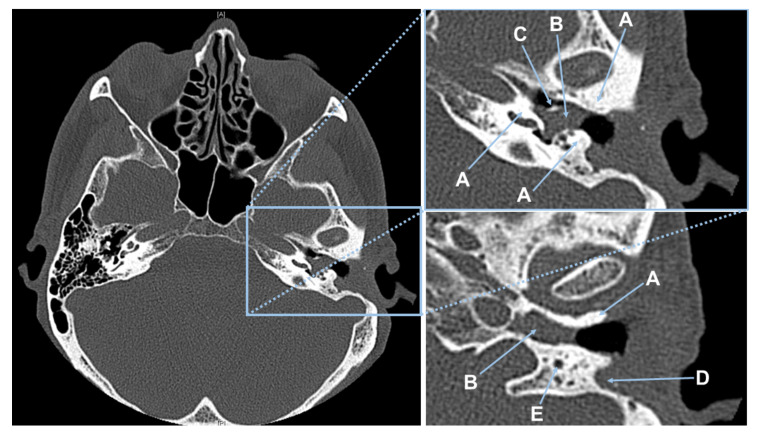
CT scan of Patient #1. (Left) Showing the complete CT slice. (Top right) Magnified CT-slice showing the patient’s left middle ear anatomy. In this view, (A) bone, (B) cholesteatoma, and (C) the handle of malleus covered with cholesteatoma. (Bottom right) Magnification of a 2nd CT-slice with (A) bone, (B) cholesteatoma, (D) connective tissue in the pre-surgical removed canal wall, and (E) the nervus facialis embedded in bone.
